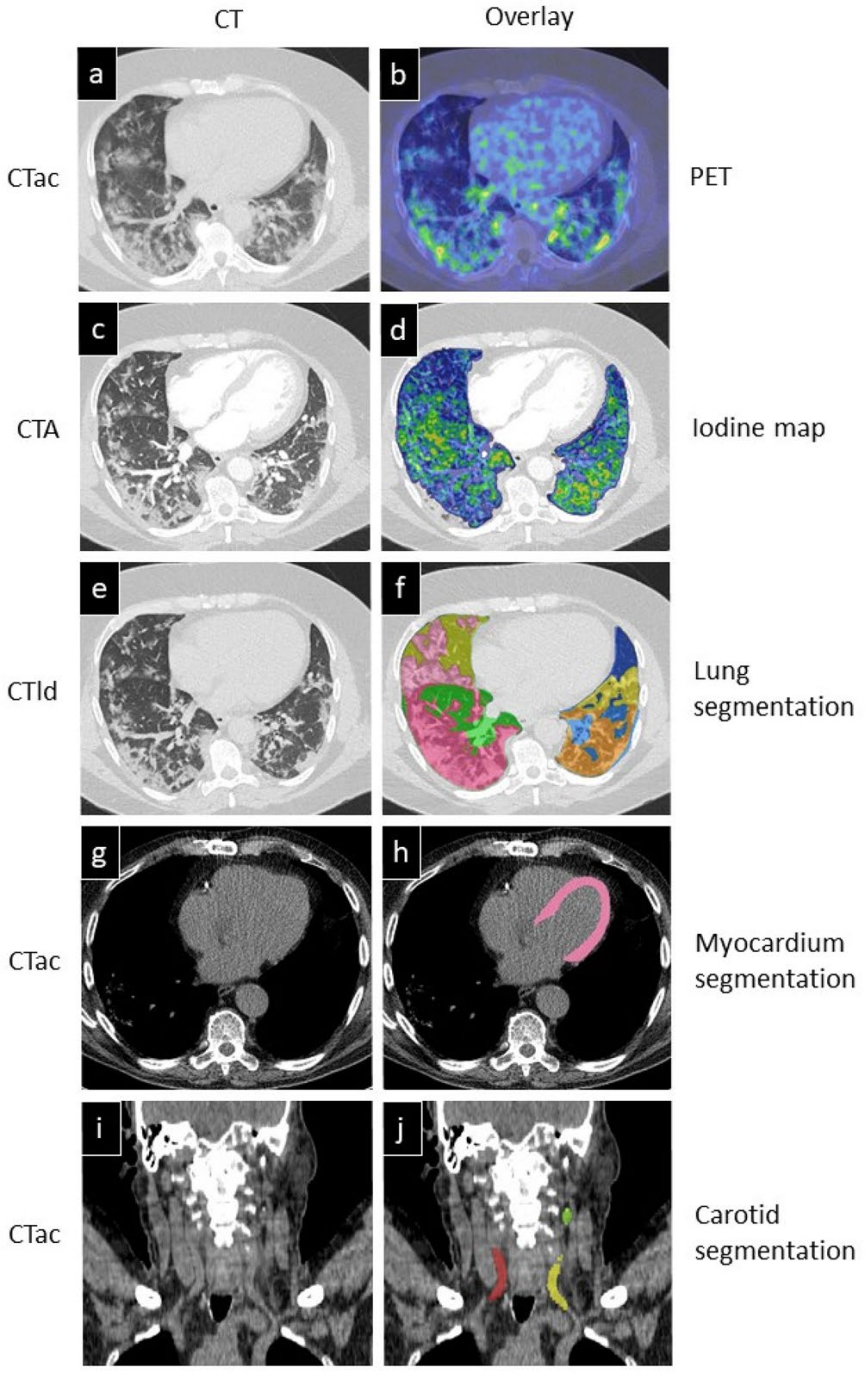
CT reconstructions with correlating PET, subtraction CT iodine mapping and segmentation overlays. (a) Axial view of CTac imaging of the thorax. (b) CTac imaging of the thorax with attenuation corrected PET overlay. (c) CTA of the thorax with breath hold after contrast injection. (d) Subtraction CT of the lungs: iodine map overlay. (e) CTld of the thorax with breath hold instruction. (f) CTld with lung segmentation overlay; Unaffected parts of lobes are colored green and blue. Lilac, pink, orange and yellow are segmented affected parts of the lobes (g) CTac imaging of the myocardium. (h) CTac with myocardium segmentation overlay. (i) Coronal view of CTac imaging of the head and neck. (j) CTac with carotid arteries segmentation overlay (red = right carotid artery, yellow = left carotid artery, green = left internal carotid artery).
